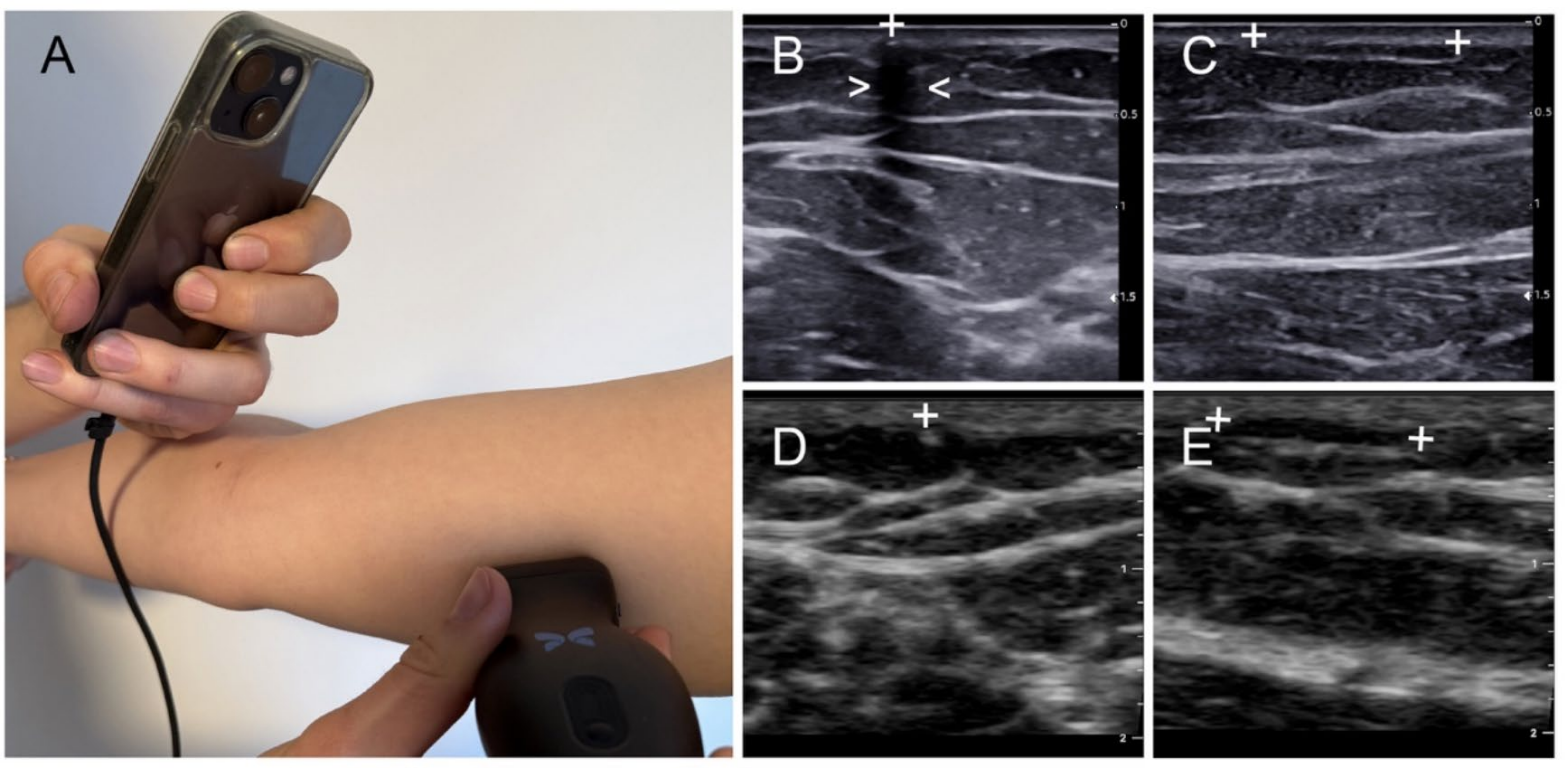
Image of an examination with a handheld ultrasound device (A) and of contraceptive implant using a standard ultrasound device based on piezo technology (B and C) and a handheld ultrasound device based on silicon chips (Dand E): The hyperechoic round region of the contraceptive implant in the transverse scan is marked by the plus and the acoustic shadow is marked by the arrow (B and D). The hyperechoic line of the contraceptive implant in the longitudinal scan is marked between the plus signs (C and E)
